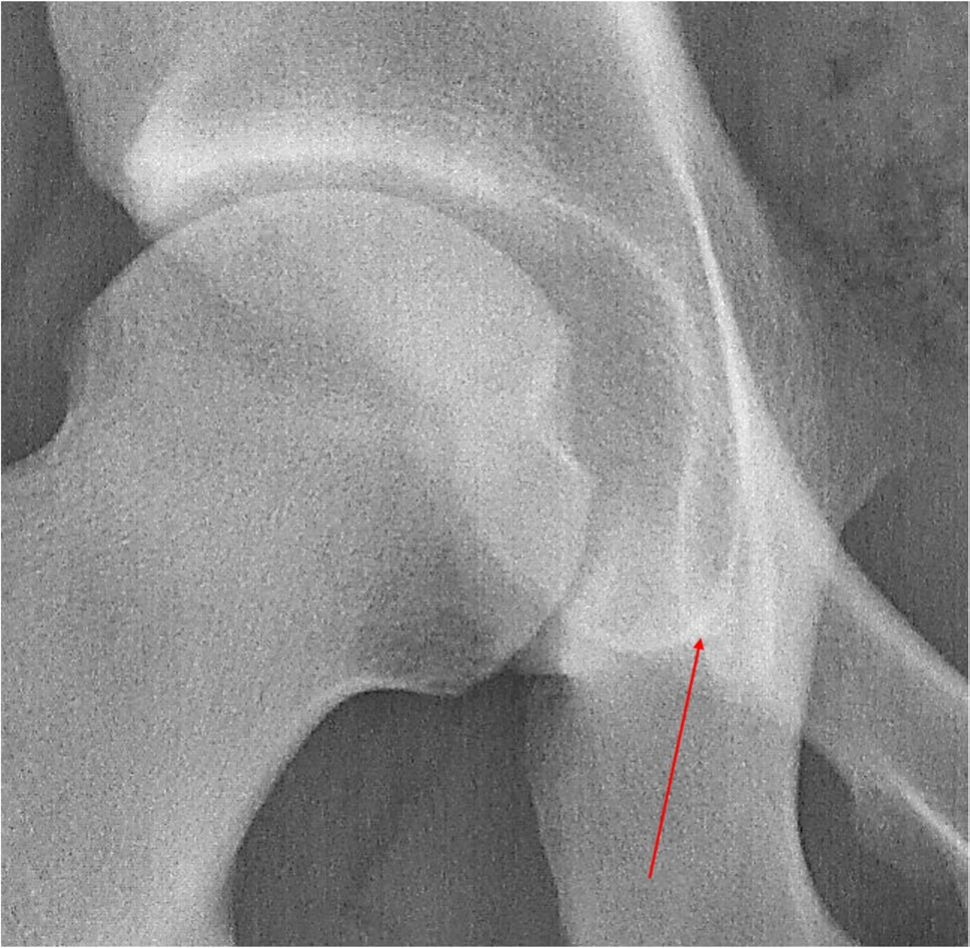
Radiograph of the right hip joint – a detail showing the “Köhler teardrop” (red arrow)
